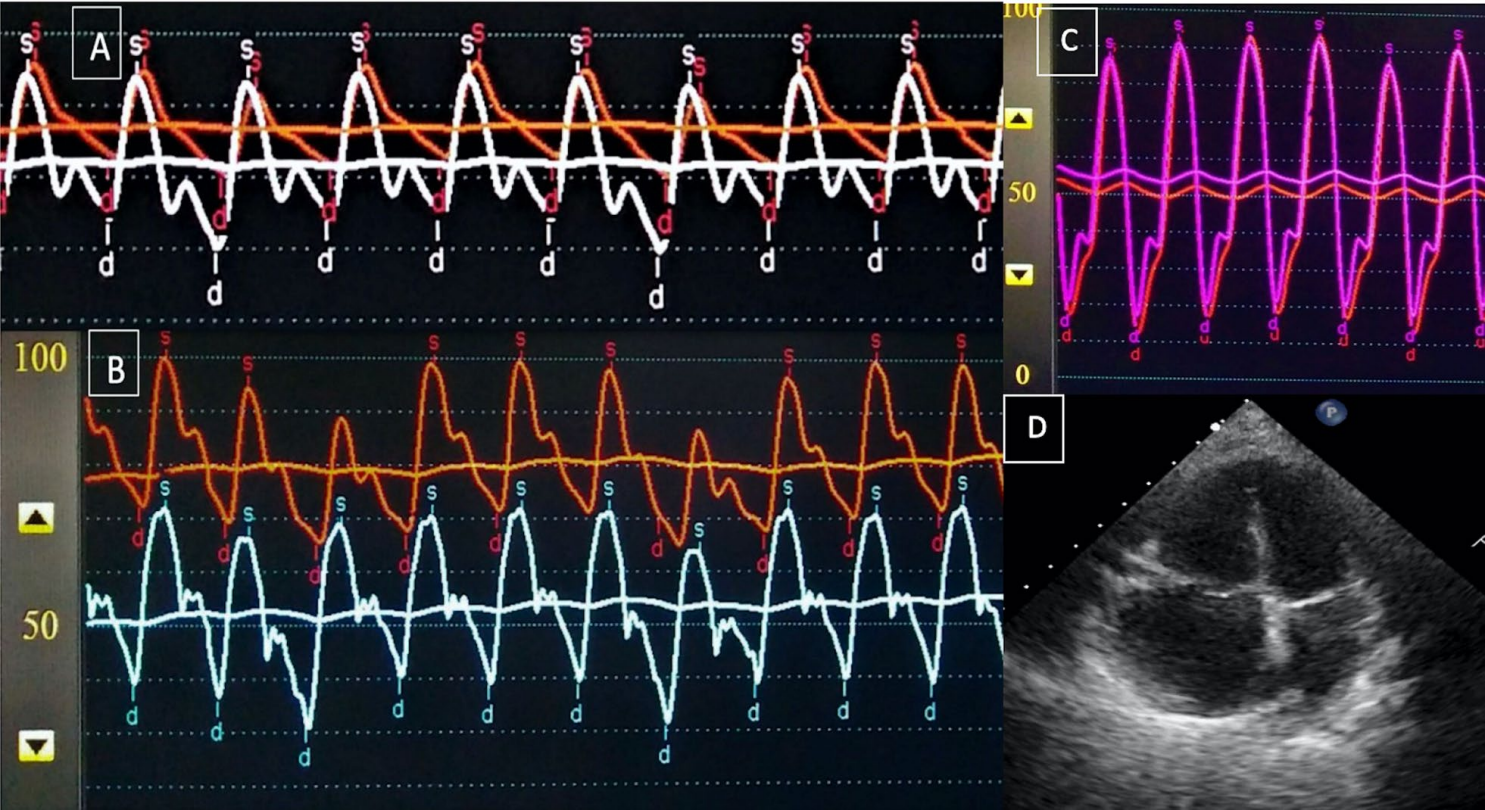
A Simultaneous aorta and pulmonary artery pressure trace at baseline B Simultaneous aorta and pulmonary artery pressure trace after 100% oxygen administration C Ventricular pressure tracing showing prominent rapid filling wave and equalisation of ventricular diastolic pressure D Echocardiography in apical four chamber view showing dilated right atrium and ventricle
